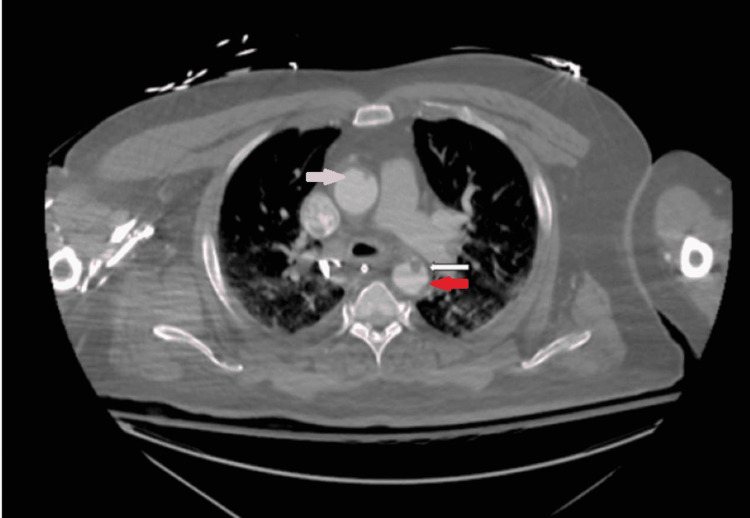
Computed tomography angiogram (CTA) of the chest revealed small filling defects in both the ascending (pink arrow) and descending thoracic aorta (red arrow), highlighted by white arrow.
